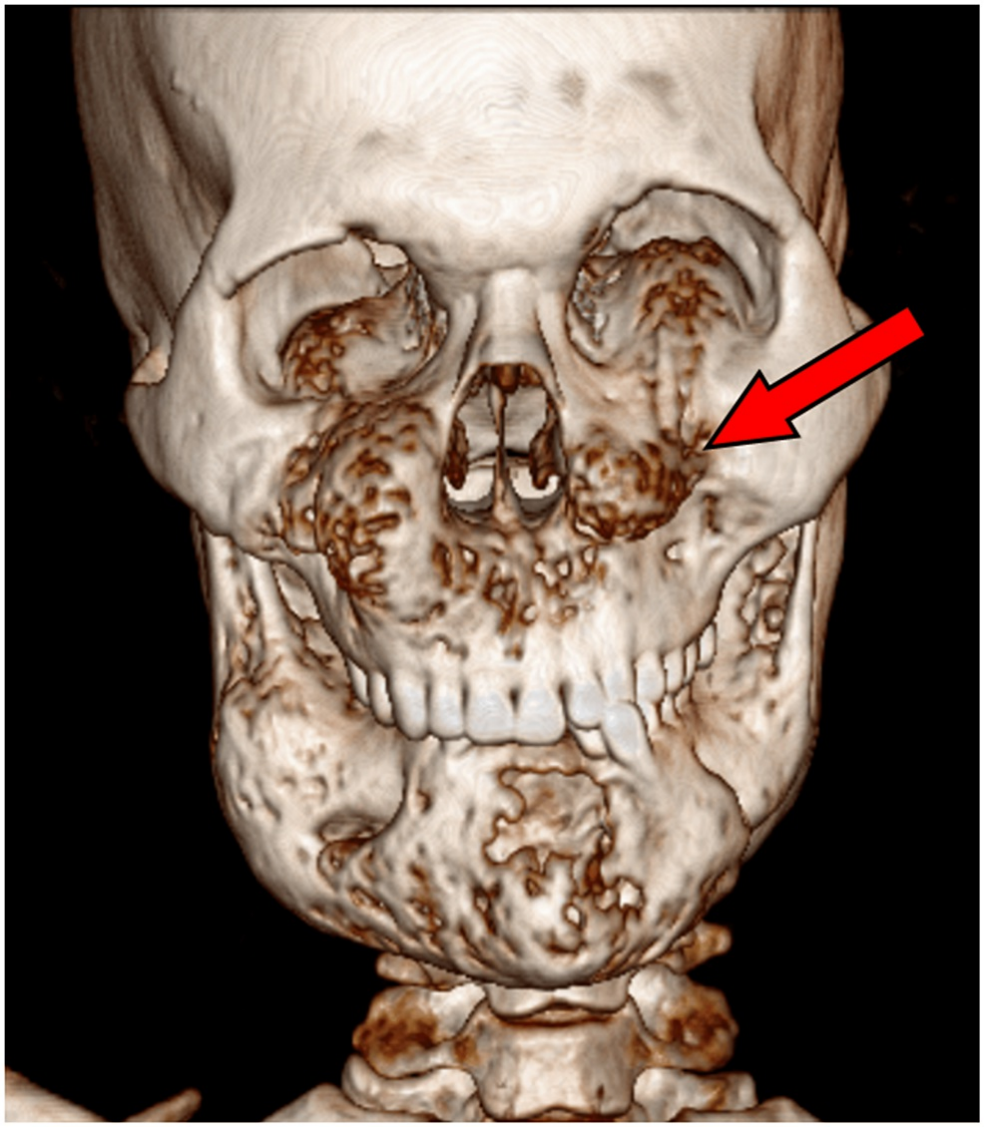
A 3D computed tomography image of a skull This figure shows the expansion of the bony calvarium with an intact cortex and loss of trabeculae, giving a homogeneous ground glass appearance noted in the mandible and maxilla, involving the bilateral floor of orbits, bilateral pterygoid plates, and maxillary sinuses, with partial obscuration of sinuses
